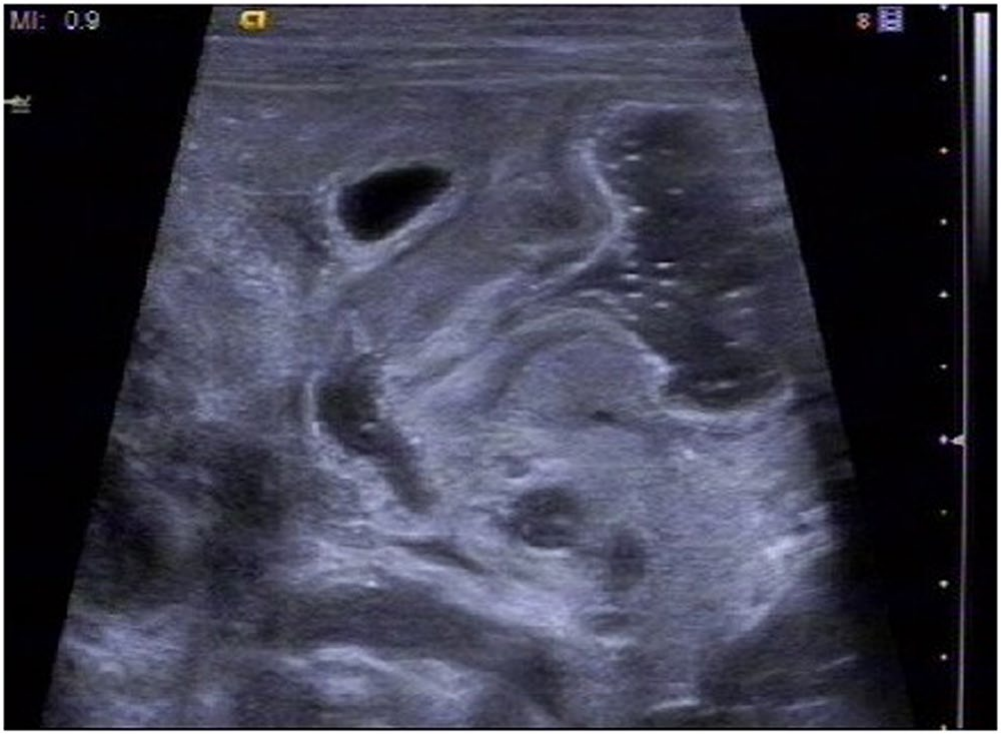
Two dimensional ultrasound for examining the liquid passing through the Pylorus.
